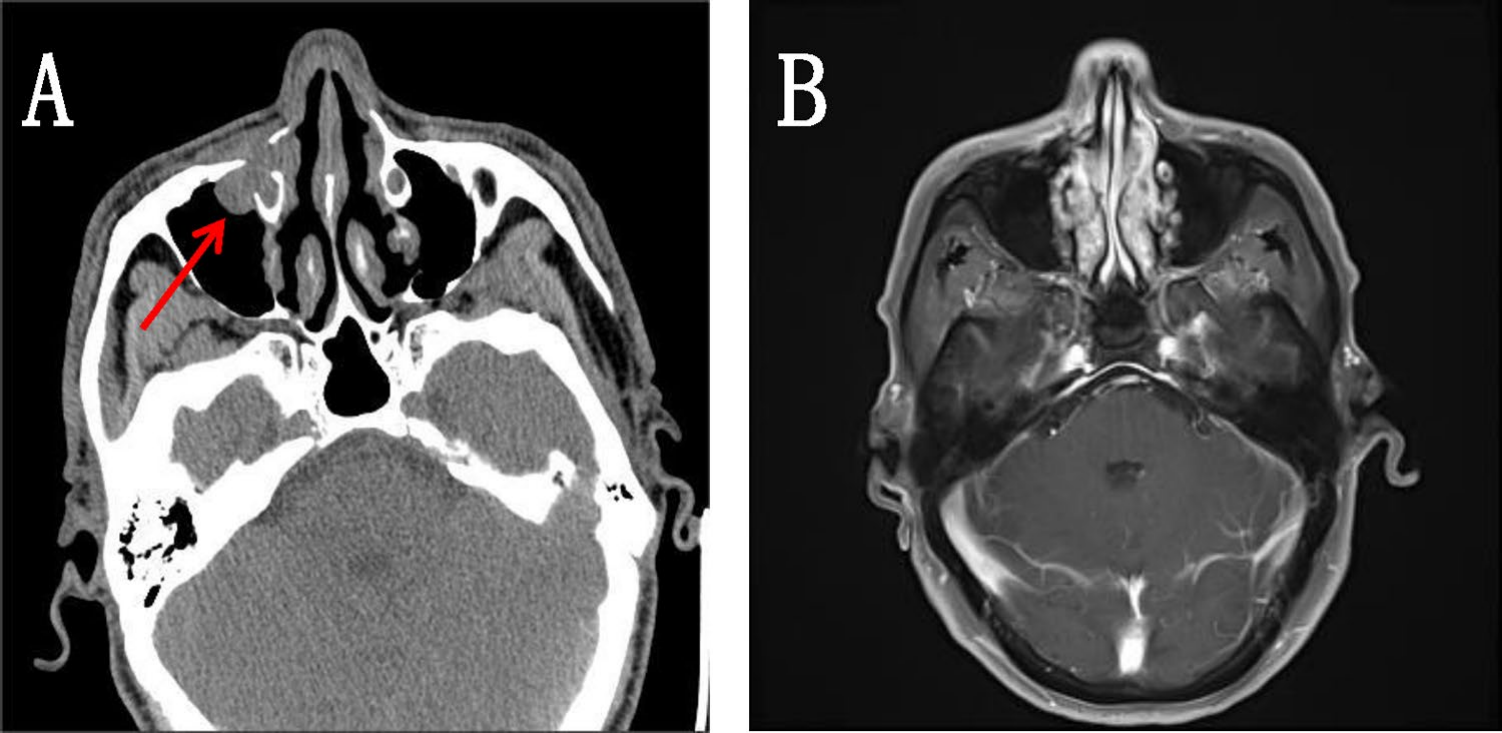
Imaging findings: A CT scan of the paranasal sinuses shows a slight soft tissue density shadow in the anterior wall area of the right maxillary sinus with adjacent bone destruction and resorption. The nasal septum is slightly deviated to the right and both nasal turbinates are enlarged. B MRI scan of the head shows: 1. Local absence of the anterior wall of the right maxillary sinus, no abnormal enhancement seen after contrast administration
